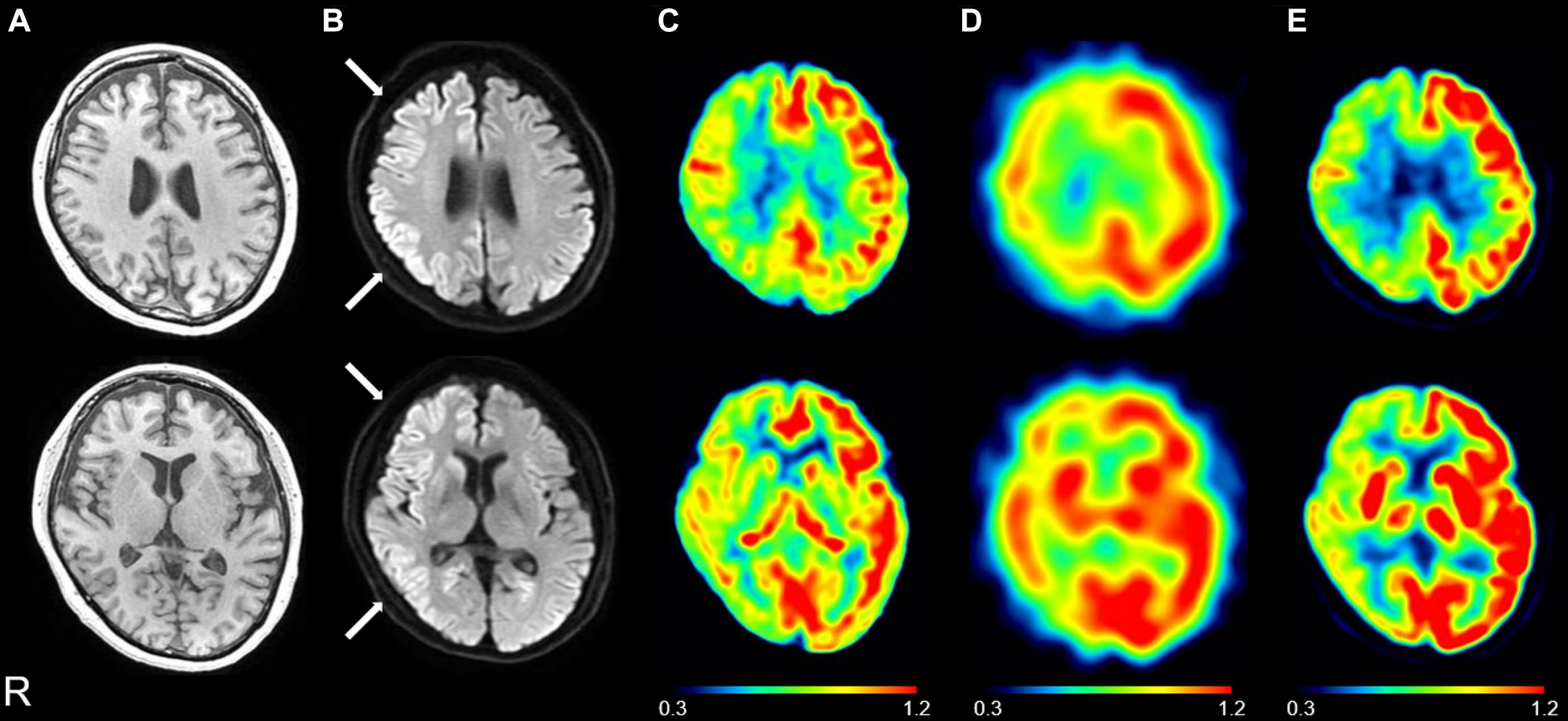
Representative images of a patient with Creutzfeldt–Jakob disease (CJD) (CJD 2: patient in their 50s). Brain magnetic resonance imaging showed normal findings on the T1-weighted image (A); the right caudate nucleus, frontal, parietal, and temporal hyperintensity on the diffusion-weighted image (B) (see white arrows); and the right caudate nucleus, frontal, parietal, and temporal hypoperfusion on the cerebral blood flow map derived from arterial spin labeling (ASL) imaging (C) on day 24. ^123^I-*N*-isopropyl-*p*-iodoamphetamine single-photon emission computed tomography (SPECT) revealed right, frontal, parietal, and temporal hypoperfusion (D) on day 28, and ^18^F-fluorodeoxyglucose positron emission tomography (PET) revealed right caudate nucleus, frontal, parietal, and temporal hypoperfusion (E) on day 29. The color bar indicates the normalized values of ASL perfusion (C), SPECT perfusion (D), and PET uptake (E).
